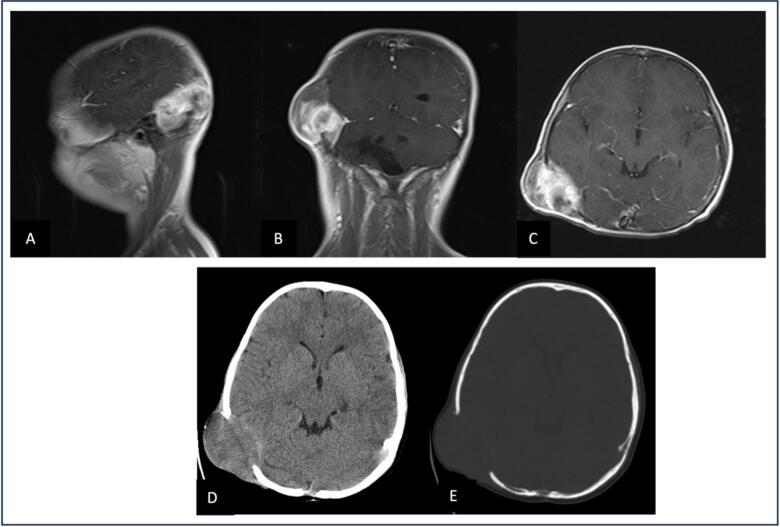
Preoperative imaging (A) T1-weighted MRI sagittal view with contrast enhancement showing a right temporo-occipital mass with heterogeneous signal intensity extending to the skull. (B) T1-weighted MRI coronal view with contrast enhancement demonstrating the extra-axial nature of the lesion with enhancement and adjacent bone involvement. (C) T1-weighted MRI axial view with contrast enhancement clearly delineating the right temporo-occipital mass. (D) CT brain window axial view revealing the soft tissue component of the mass with isodense appearance. (E) CT bone window axial view highlighting the lytic bone lesion with destruction of the right temporo-occipital bone.
